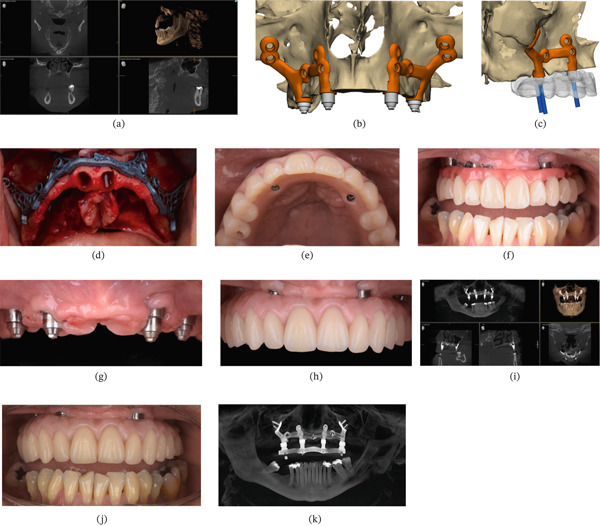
Clinical presentation of Case 2. (a) CBCT scan of Patient 2 showing a severely atrophic edentulous maxilla. (b and c) Three‐dimensional reconstructions of the subperiosteal implant design for the upper jaw and digital planning. (d) Mucoperiosteal flap incised and elevated and custom‐made cutting guide in place using 2.0 mini screws. (e) Occlusal view of the temporary prosthetic immediately screw‐retained prosthesis based on a 3D‐printed design. (f) Frontal view of the immediate temporary prosthetic screw‐retained prosthesis in Patient 2. (g) Temporary immediate restorations were removed after healing for 4 months. (h) Intraoral frontal view of the final prosthesis. (i) CBCT was performed to evaluate the accuracy of the prosthesis stability and the surrounding bone. (j) Intraoral clinical image of the definitive prosthesis at the 42 months follow‐up. (k) Panoramic radiograph of the patient taken at follow‐up.
